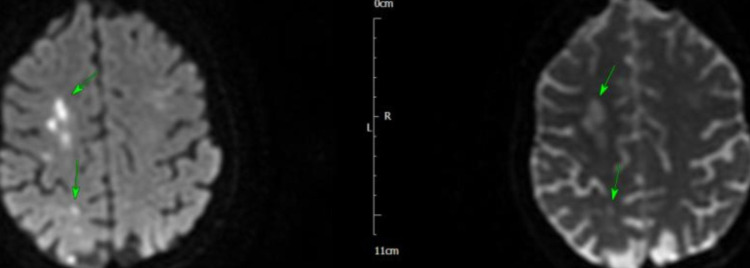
MRI of the head showing haemorrhagic infarcts (arrows)
